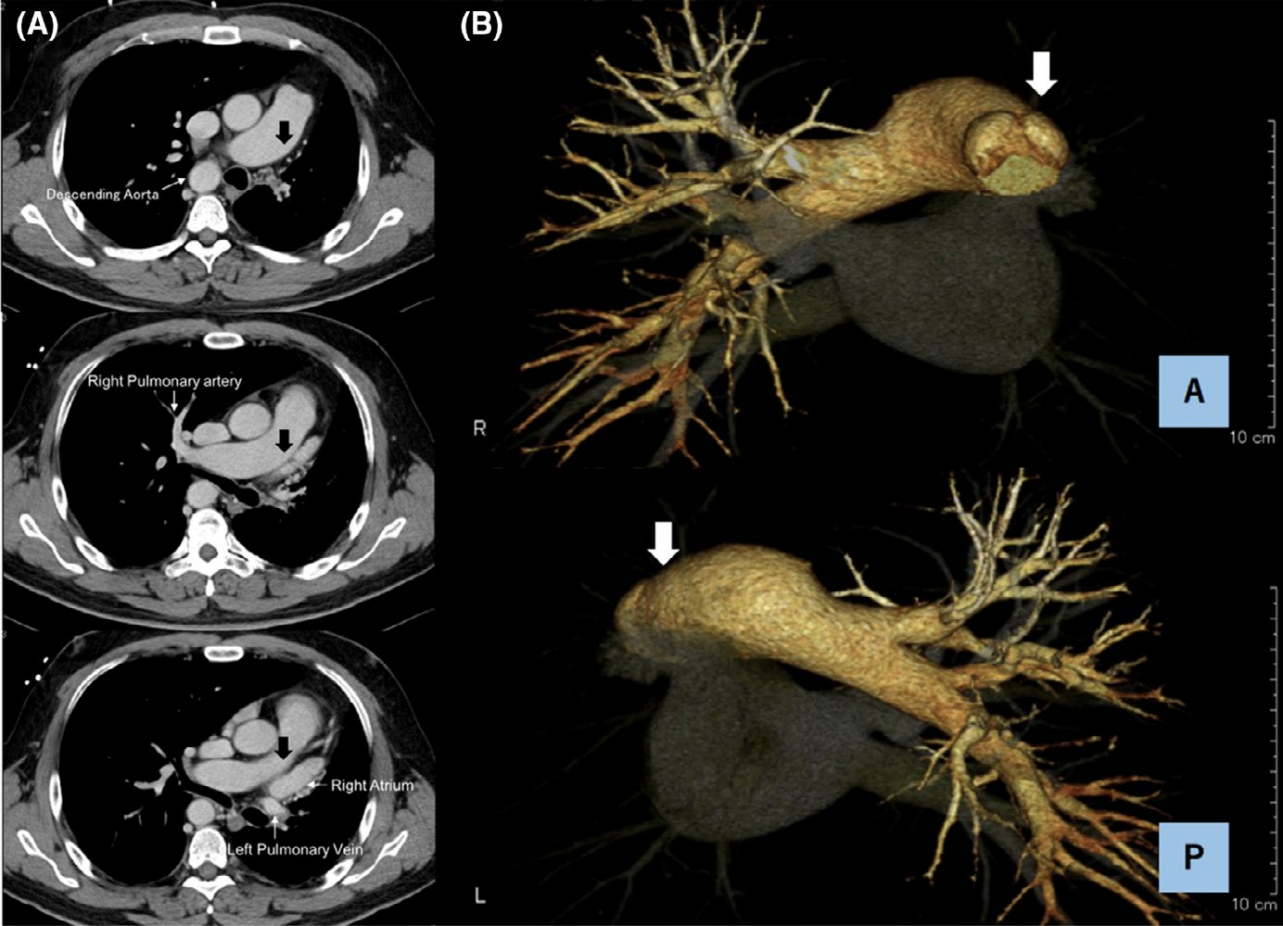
Preoperative images. A, Contrast‐enhanced computed tomography (CT) shows the absence of the left pulmonary artery (black arrows). B, Three‐dimensional CT clearly shows the absence of the left pulmonary artery from its origin in an anterior view and posterior views (white arrows)
